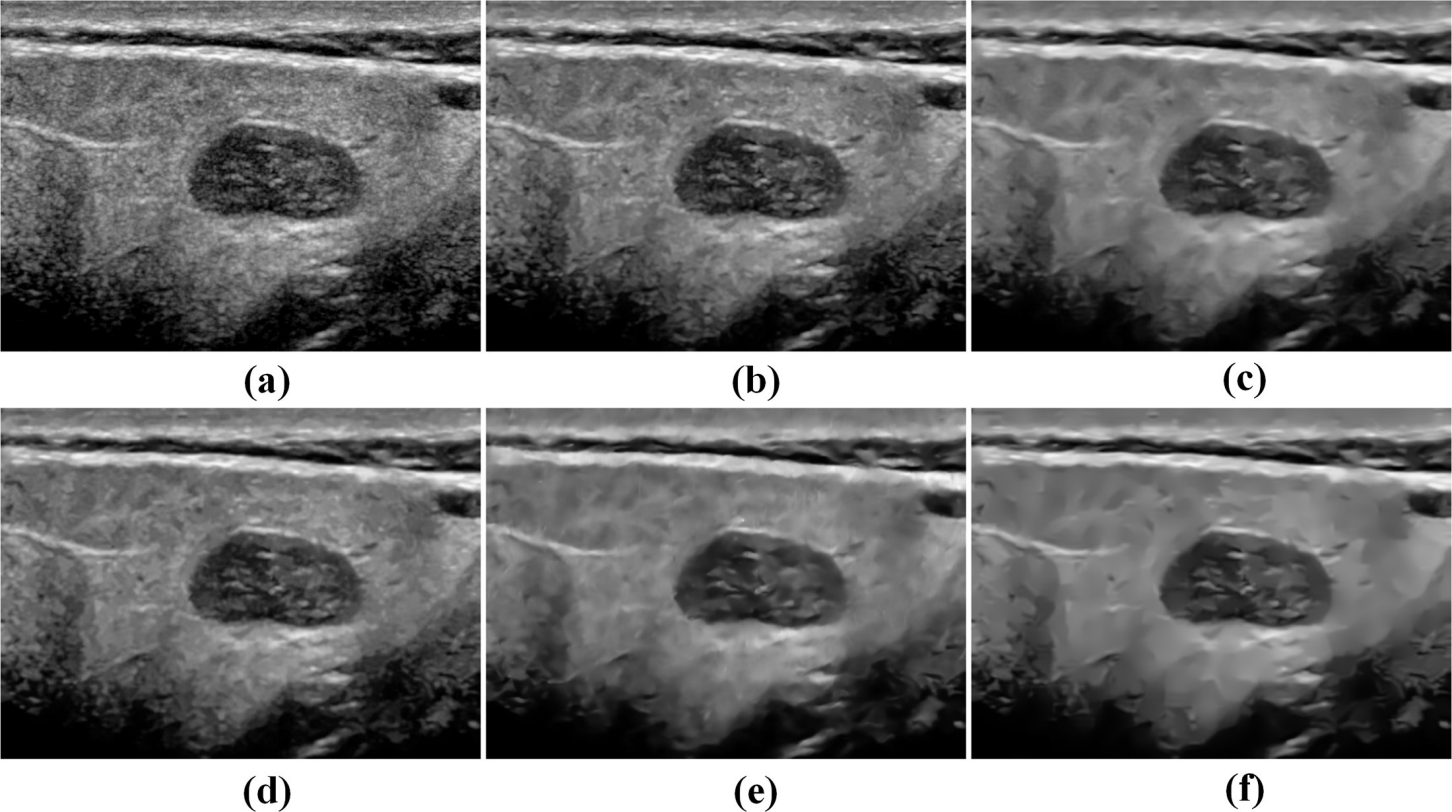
Comparison of restoration performance on a real parotid gland adenoma ultrasound image for the NLM-based and DnCNN methods. (a) original image, (b) TNLM filter, (c) OBNLM filter, (d) NLMLS filter, (e) DnCNN filter, and (f) PPCA-NLM filter.
